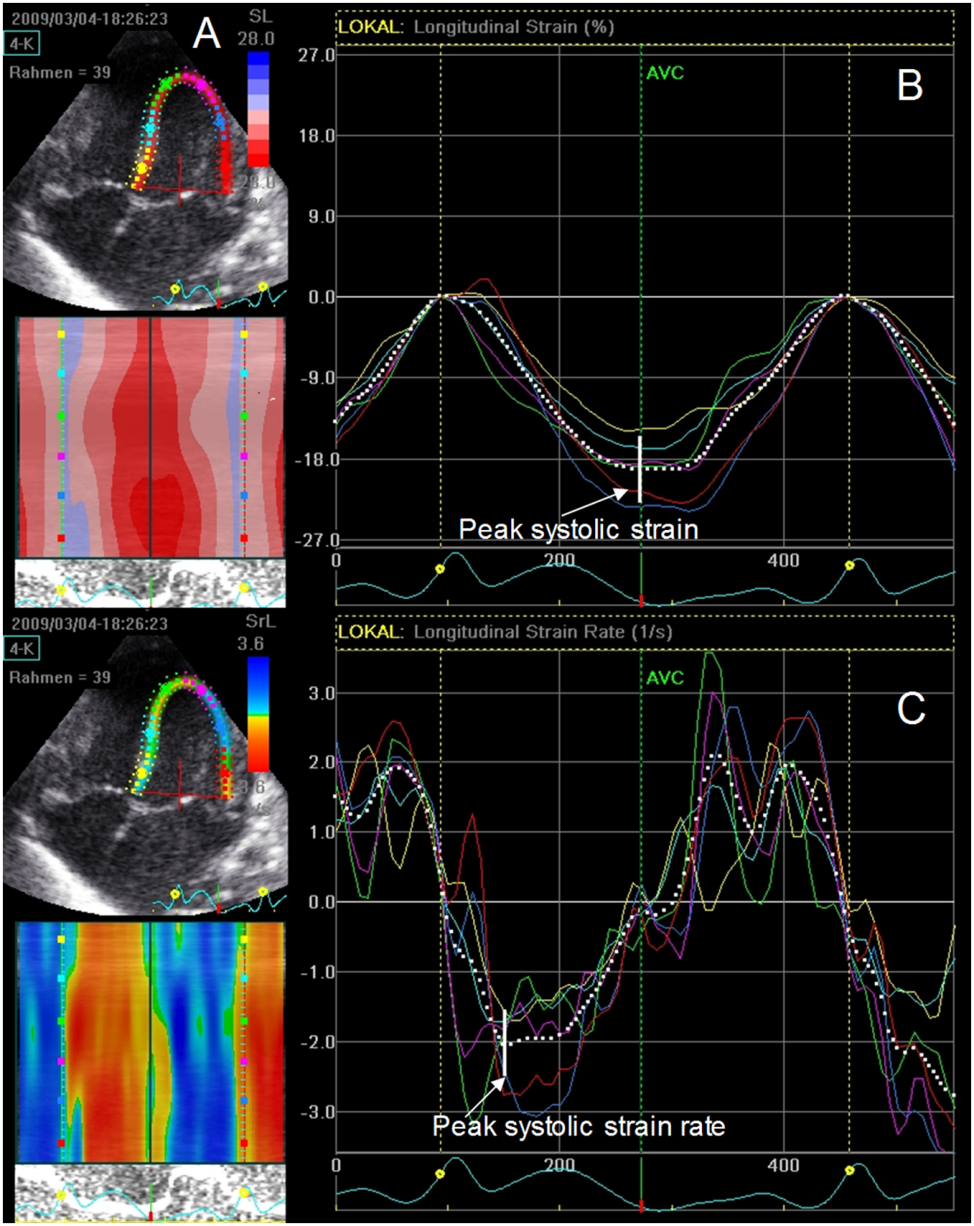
Measurement of peak systolic strain and strain rate. (A) 2D apical four-chamber view of the left ventricle with region of interest (in color with each color representing one of six segments) encompassing endo- and epicardium for 2D strain and strain rate analysis. (B) Global longitudinal strain (white dotted line) was calculated by averaging measurements of six segments in the apical four-chamber view. (C) Global longitudinal strain rate (white dotted line) was calculated by averaging measurements of six segments in the apical four-chamber view.
